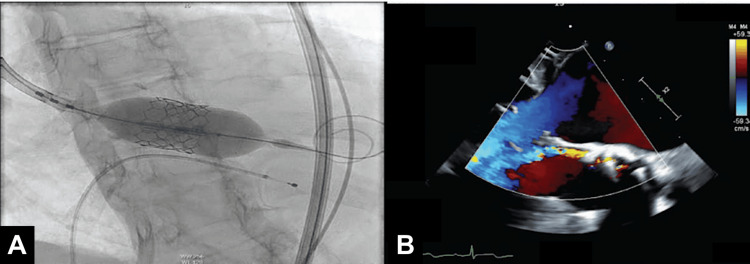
(A) A 23 mm Edwards Sapien 3 Ultra Resilia valve deployed. (B) A transesophageal echocardiogram (TEE) reveals a moderate to severe aortic paravalvular leak (PVL) following transcatheter aortic valve replacement (TAVR). Figures reprinted from [12], ©2025 HMP Global. Used with permission.
